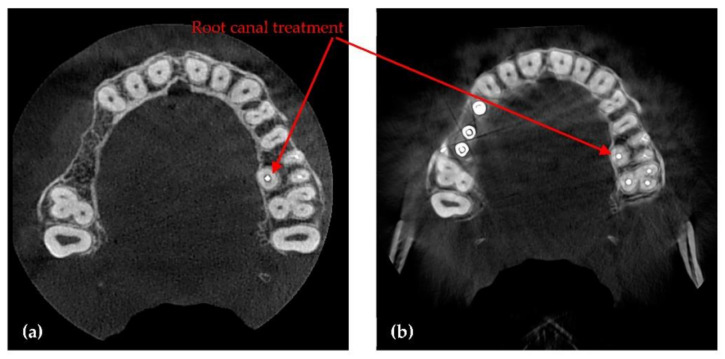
Example of an axial section (a) before and (b) after the optimization of the 3D CBCT imaging.
